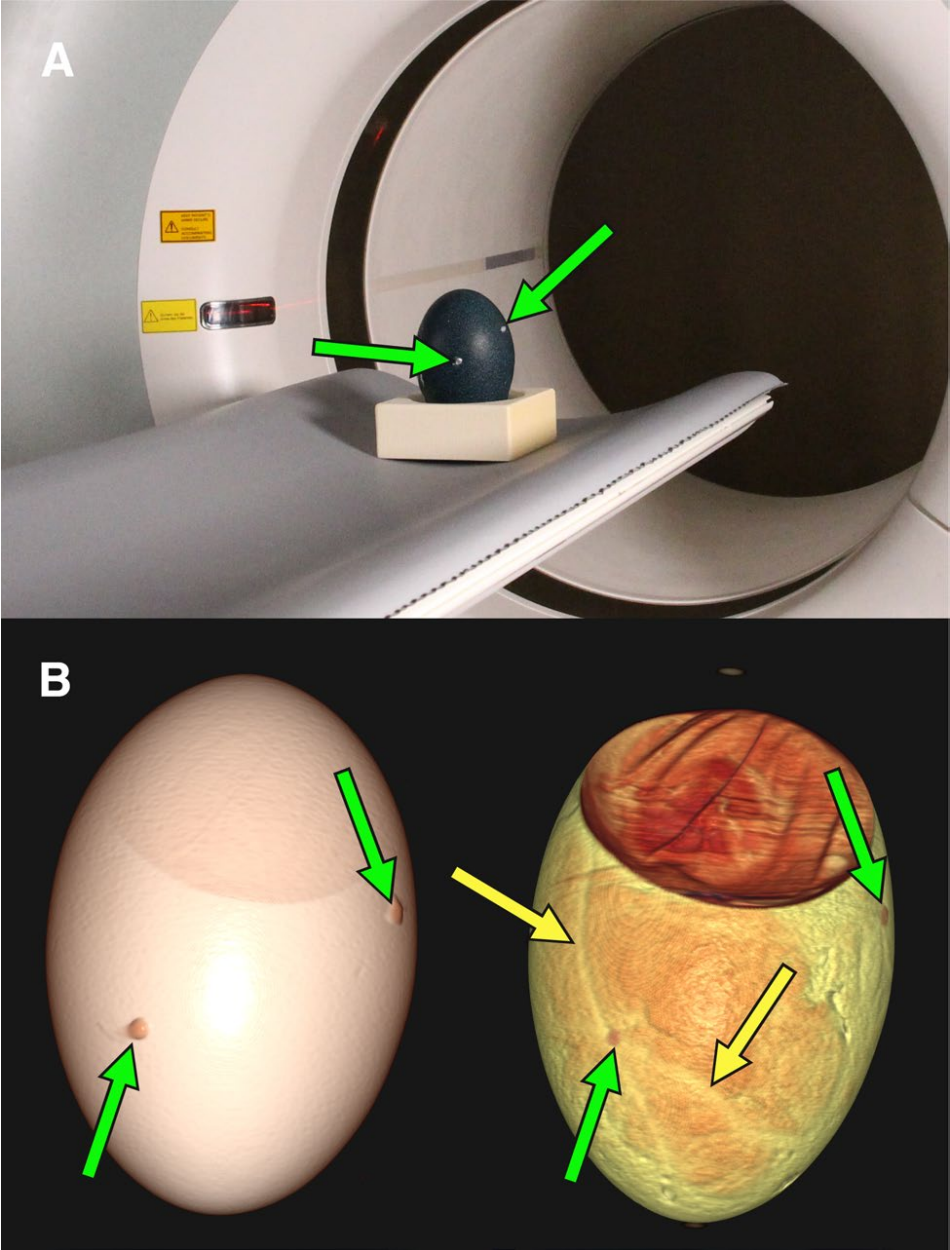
(A) Positioning of an emu egg for CT scan. (B) Three-dimensional reconstruction in VRT shows the eggshell (left side) with CT markers (green arrows) and blood vessels (yellow arrows) beneath the eggshell (right side) (Created in https://BioRender.com)
